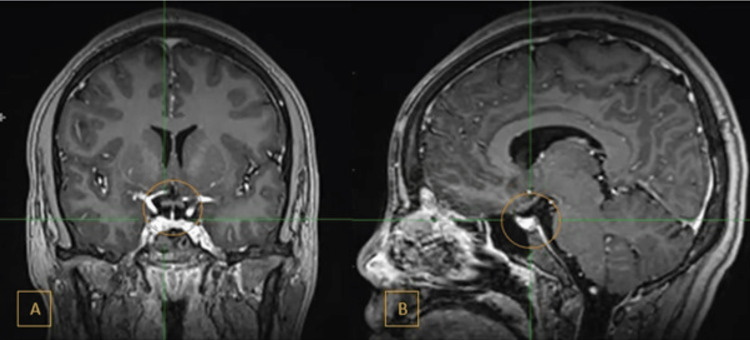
Contrast-enhanced T1-weighted MRI (A) Coronal view. (B) Sagittal view. Orange circle: normal pituitary gland, suprasellar cistern, and optic chiasm MRI: magnetic resonance imaging
